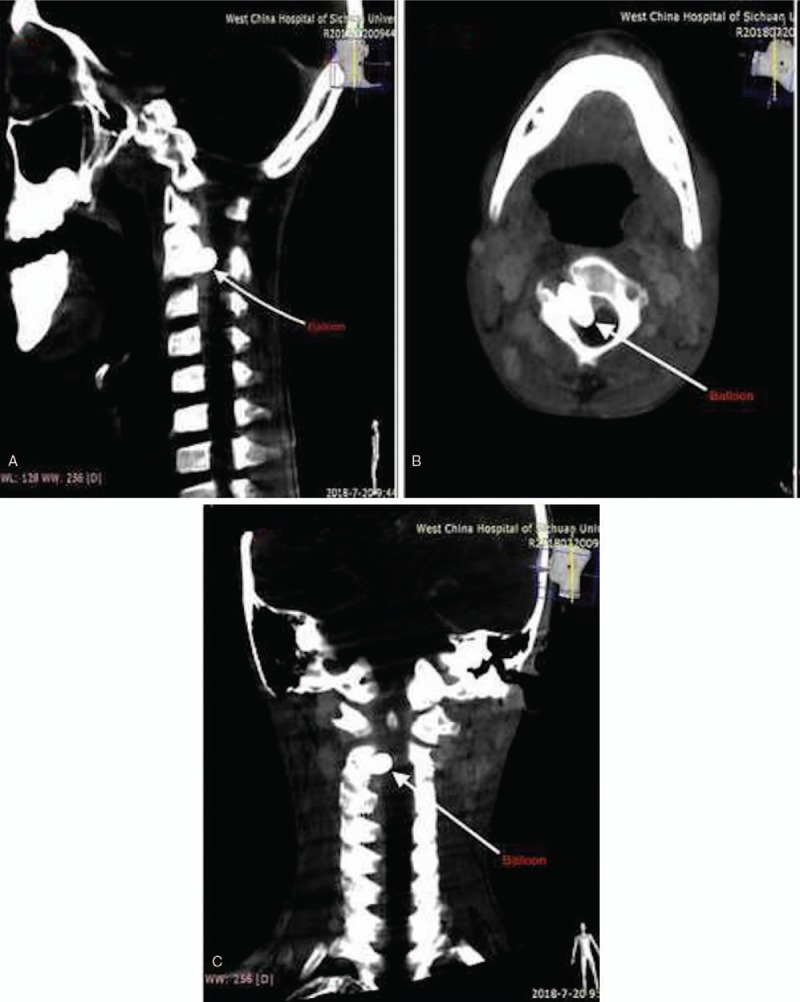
A, B, and C are postoperative magnetic resonance imaging images showing occlusion of the fistula with the balloon.
